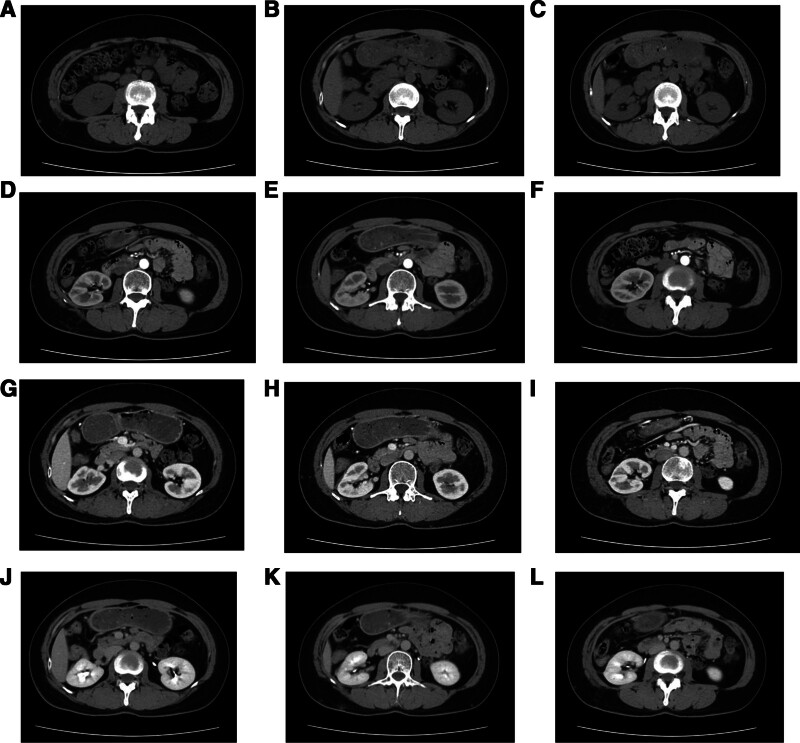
Four-year postoperative surveillance with non-contrast and contrast-enhanced renal CT. CT = computed tomography.
